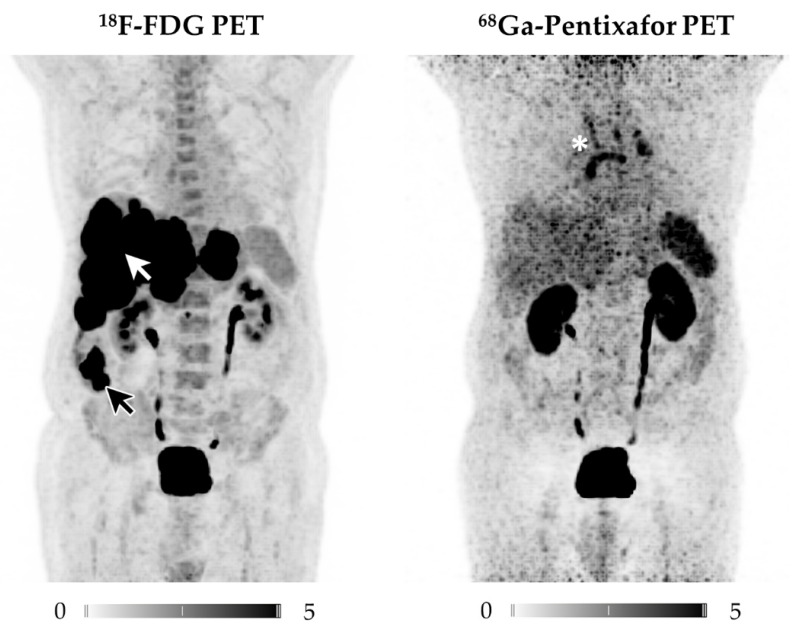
Displayed are Maximum Intensity Projections (MIP) of the ^18^F-FDG (left) and ^68^Ga-Pentixafor PET scans of patient #2. Whereas ^18^F-FDG depicts the ileal primary (black arrow) as well as multiple liver metastases (white arrow), none of the tumor manifestations are revealed by CXCR4-directed PET imaging. Incidental finding: The mediastinal tracer uptake in ^68^Ga-Pentixafor PET (white star) was traceable to enlarged mediastinal lymph nodes, most likely due to chronic lung fibrosis and not related to NEC, as follow-up imaging confirmed.
